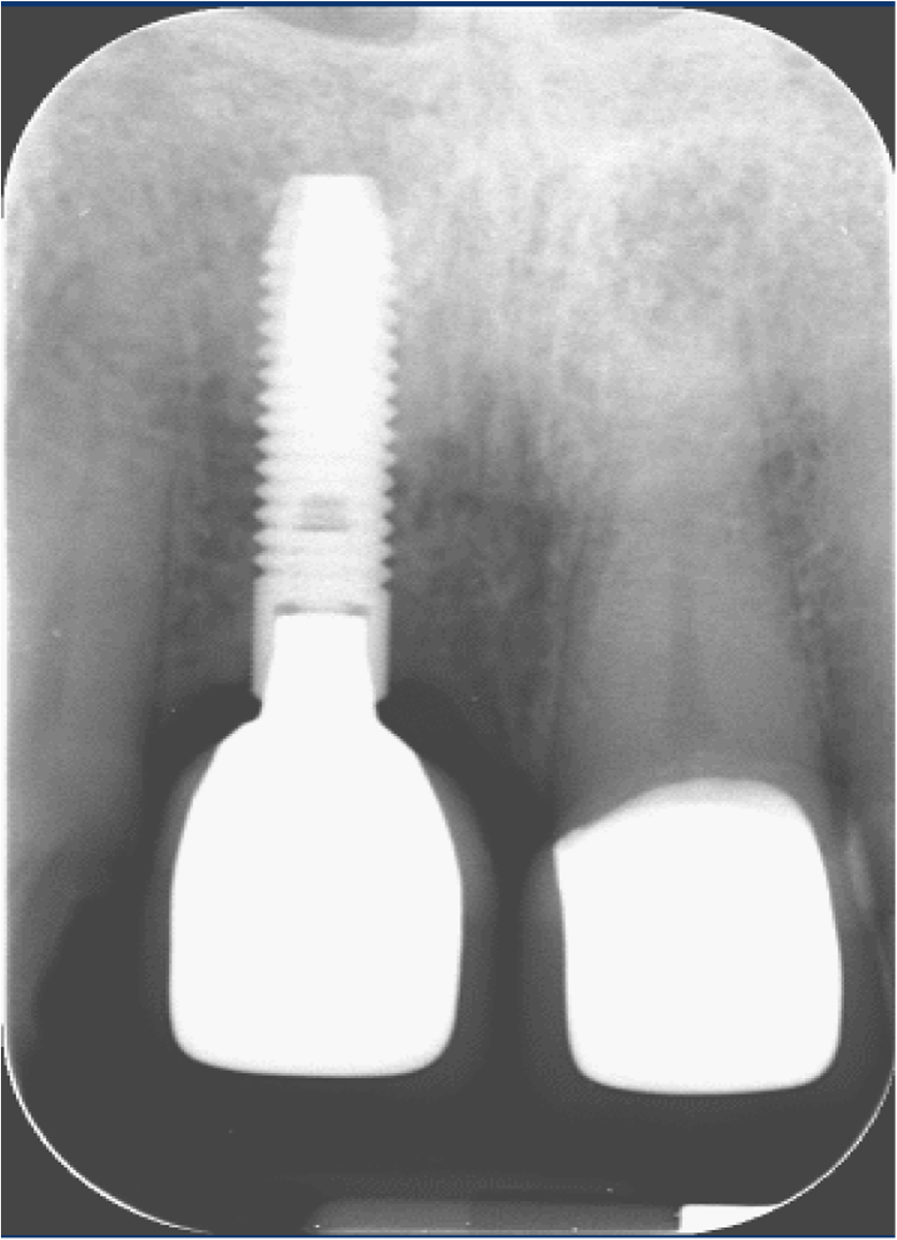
Single tooth X-ray, 1 year post-implantation, showing the finalized crown
